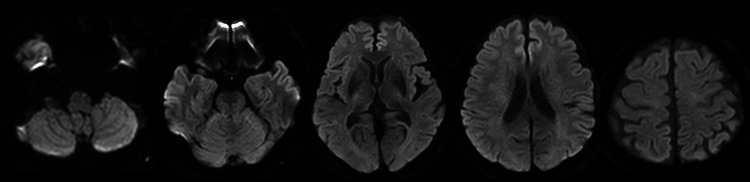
Diffusion-weighted magnetic resonance images showing no acute ischemic or hemorrhagic lesions
